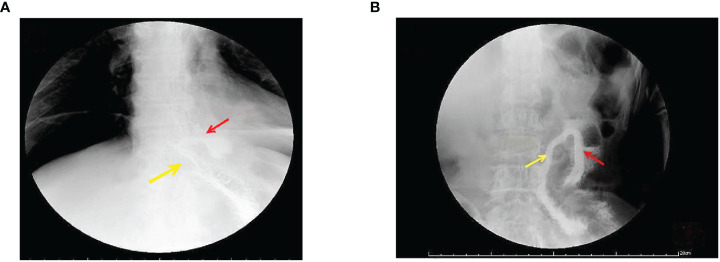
Gastrointestinal angiography at 1 week postoperative. (A) Radiographic examination of the digestive tract 1 week postoperatively. The yellow arrow indicates a patent outflow loop at the esophagojejunal anastomosis, and the red arrow points to the non-dissected closed blind end, showing no recanalization. (B) Radiographic examination of the digestive tract 12 months postoperatively. The yellow arrow indicates a patent outflow loop at the esophago-jejunal anastomosis, and the red arrow points to the non-dissected closed blind end, showing no recanalization.
